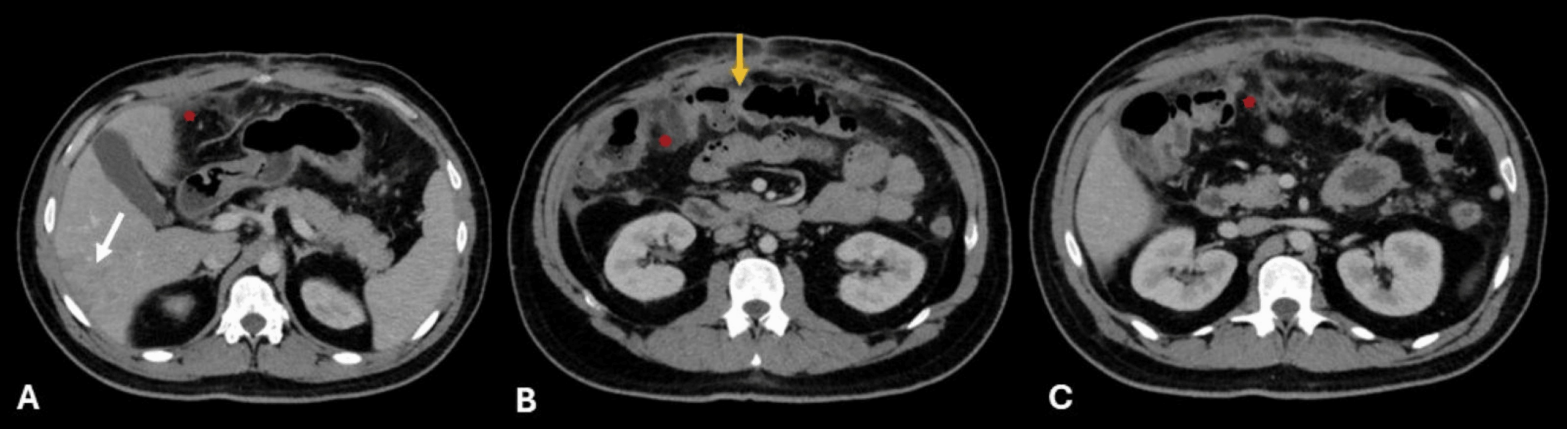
Axial contrast-enhanced abdominal CT A) Reduced size of right hepatic lobe abscesses, now with heterogeneous enhancement but without fluid (white arrow). B) Resolution of colonic wall inflammatory changes with normal wall thickness and enhancement (yellow arrow). A-C) Improved mesenteric fat stranding and laminar ascites (red stars).
